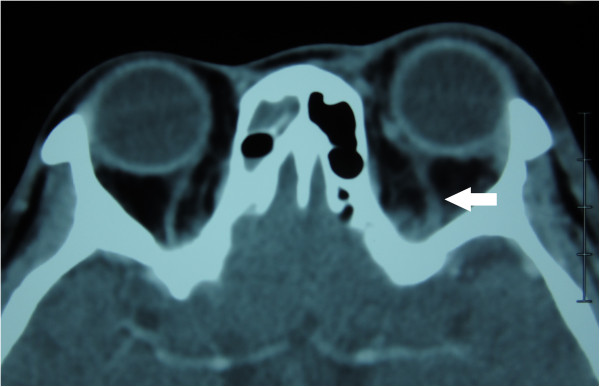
Contrast-enhanced CT. CT shows dilation of the left superior ophthalmic vein (arrow).
